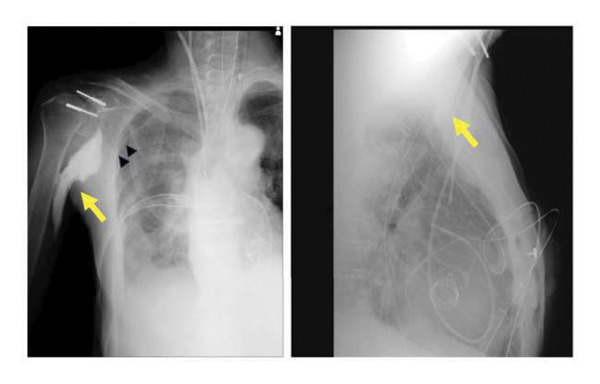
Postoperative frontal and lateral chest radiographs showing contrast medium spreading from the serratus anterior plane block catheter. The contrast medium (yellow arrow) is seen extending from the chest wall (second to fourth ribs), detached from the thorax, toward the right brachium. The fourth rib is marked with black arrowheads.
